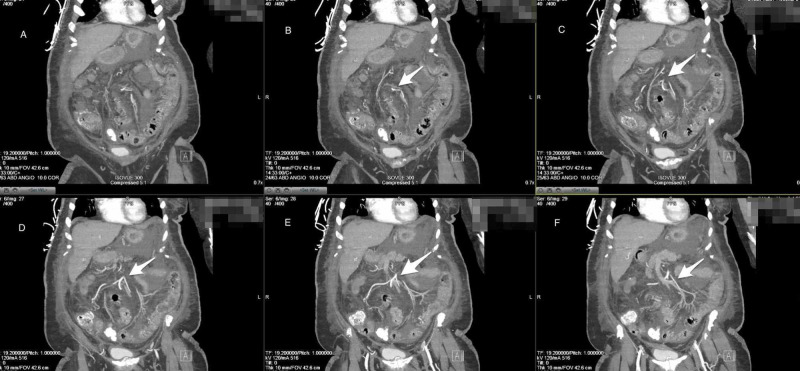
CT Angiography Abdomen and Pelvis (coronal plane) A-F) Showing no filling defects in the mesenteric arteries and only moderate atherosclerotic plaque formation of the same vessels
